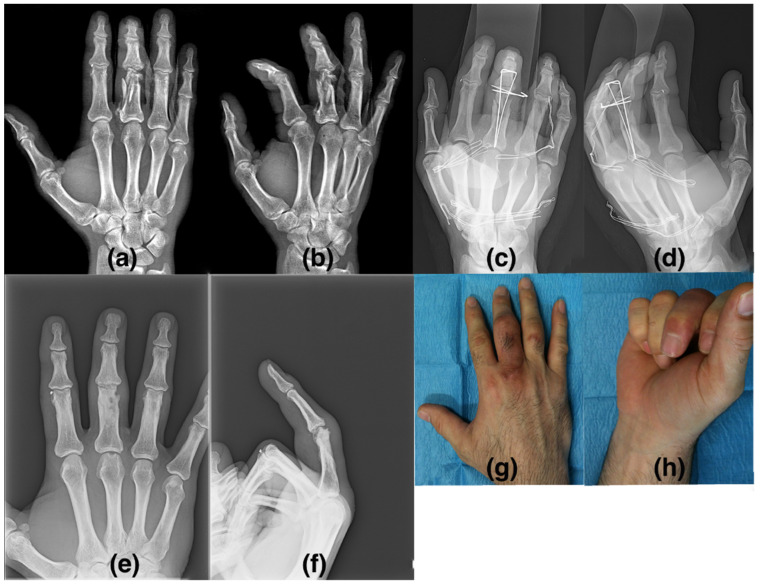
This figure shows clinical example of complex fracture treated in emergency theater that undergone biophysical stimulation. (a,b) show hand X-ray; we can notice the multiple fragmented fractures in anteroposterior view (a) and oblique view (b); (c,d) show X-ray image at T1, consisting of biophysical stimulation prescription; (e,f) show fracture healing at T6 in X-ray anteroposterior view (e) and later lateral view; (g,h) show good clinical result.
